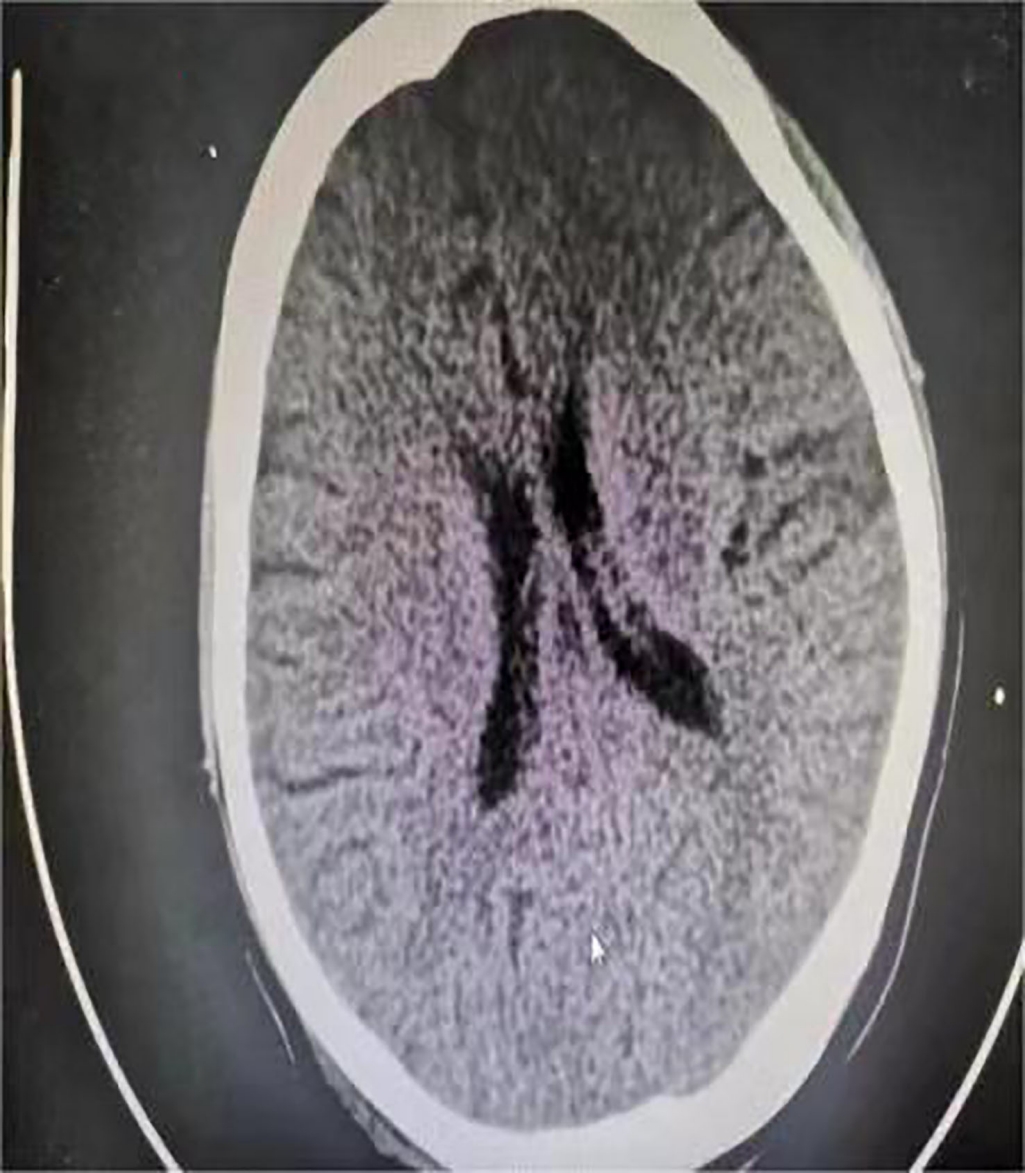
Computed tomography of the patient's head after cesarean section.
